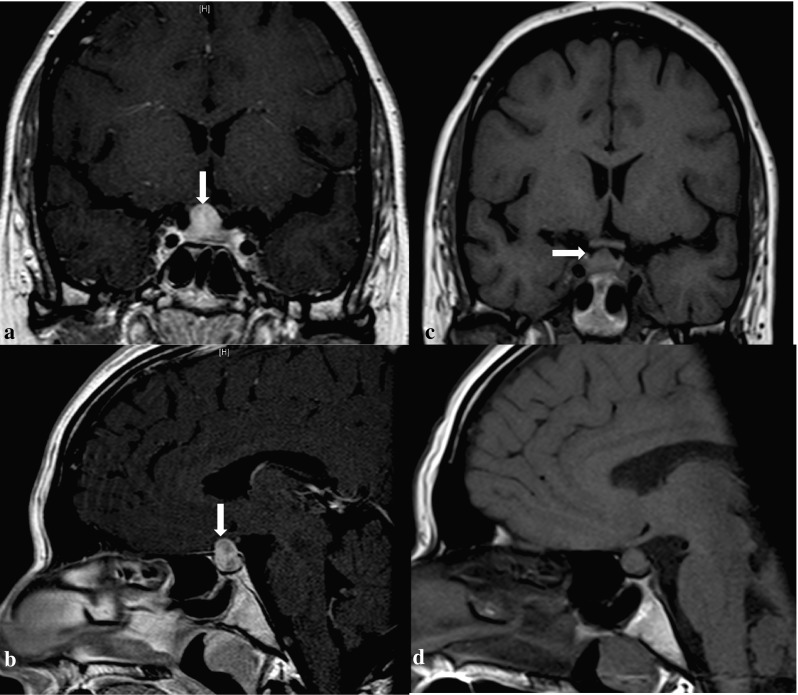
T1-weighted MRI (coronal and sagittal view) of a of 32-year-old woman (Case 3) with GPA associated PD showing a sellar mass with homogenous enhancement before (a and b) and after (c and d) treatment
